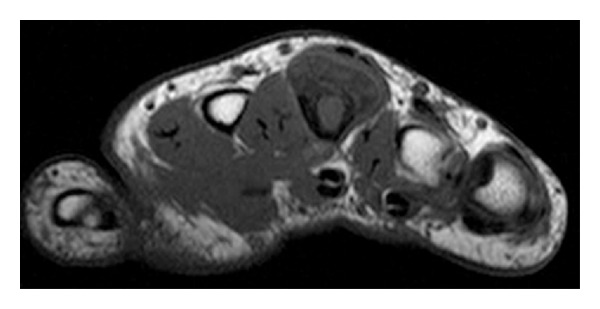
On an axial T1-weighted preoperative MR image of the area, the lesion is composed predominantly of central signal isointense to that of the normal bone marrow with a thin low signal periphery consistent with the cortical bone. Direct continuity with the underlying marrow of the bone is not noted.
